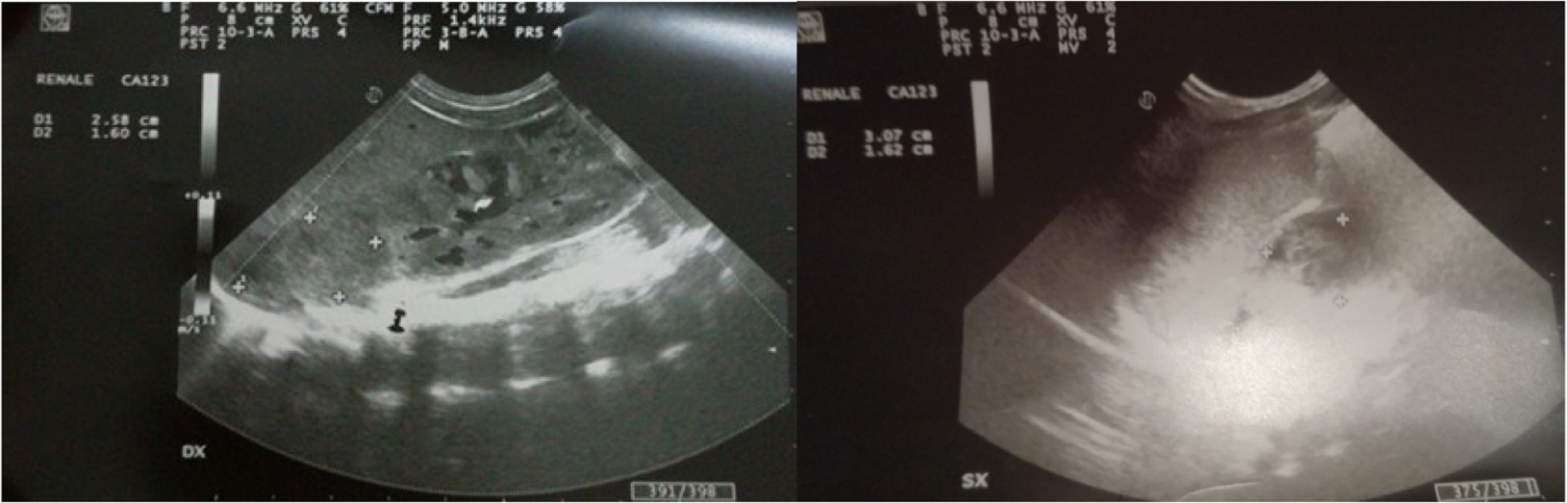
Abdominal ultrasonography revealed suprarenal bilateral lesion, well circumscribed with an inhomogeneous aspect but without vascular flow on Color-Doppler images. The images (25 mm × 16 mm right, 30 × 16 mm left) were mostly isoechoic- hyperechoic. There was a solid portion with essentially normal kidneys and no foci of blood flow within the area
